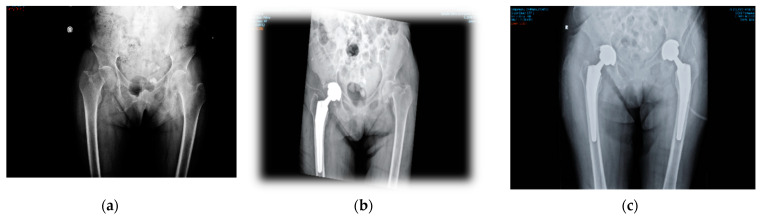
Case 1: X-ray radiographs. (a) The R femoral neck fracture is visible initially, but not the L one. (b) R femoral neck fracture reduced with prosthesis. The L crack is revealed in surgery. (c) The L femoral neck fracture is fixed with prosthesis seven days post R-side surgery.
